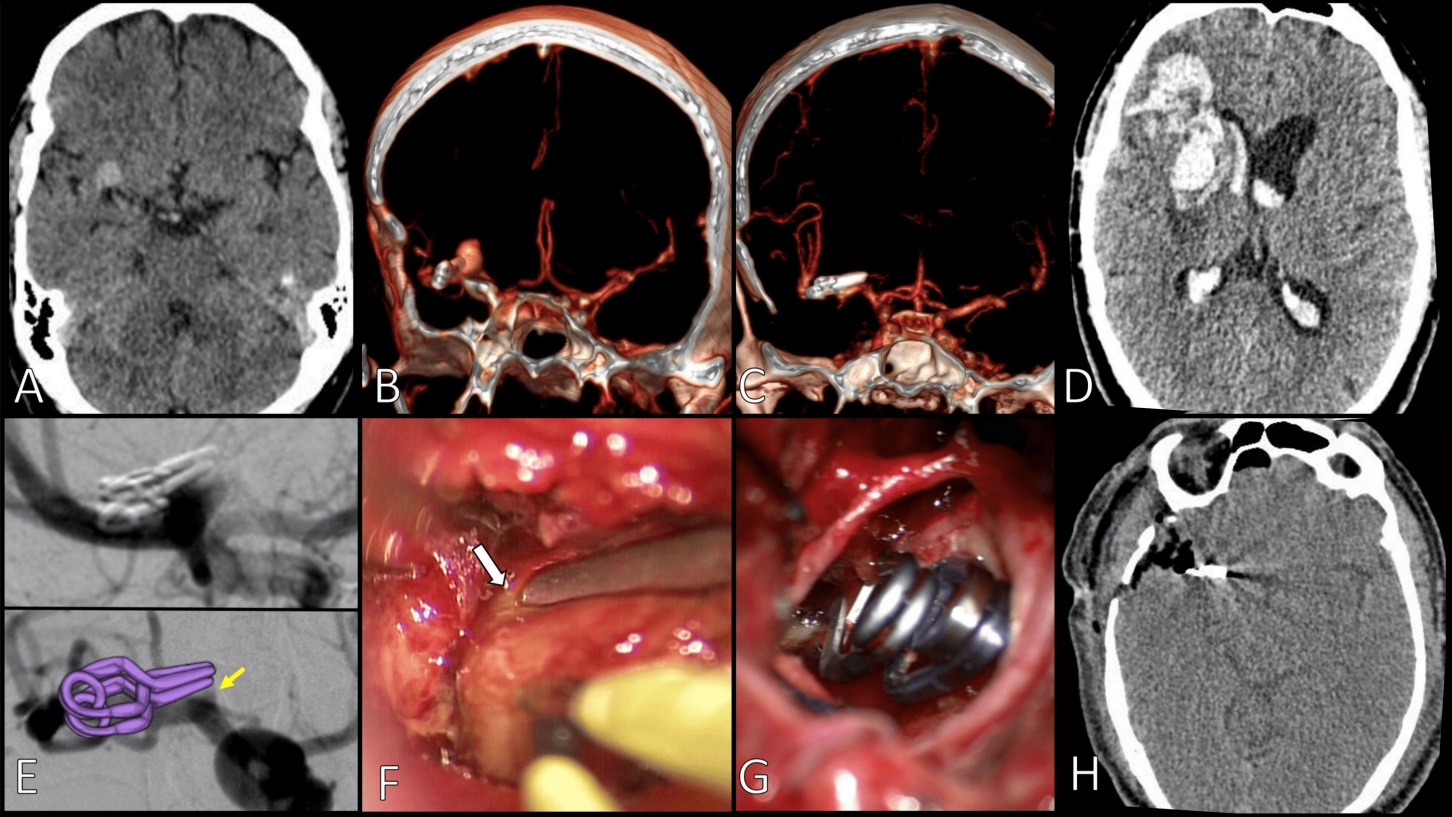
Case 1. (A) CTA showing a small hematoma in the right MCA bifurcation territory. (B) A 3D reconstruction showing an old clip dating from 2004 at the right MCA with a recurrent aneurysm. (C) No evidence of remnant aneurysm. (D) CT scan with a new SAH Fisher 4. (E) DSA images, (up) clip position enhancement (low), show a small residual at the base of the neck (arrow). (F) A remnant aneurysm was observed ahead of the clip tip (arrow). (G) Final view after re-clipping. (H) Postoperative CT scan MCA: middle cerebral artery; CTA: computed tomography angiography; DSA: digital subtraction angiography; 3D: three dimensional; SAH: subarachnoid hemorrhage; CT: computed tomography
